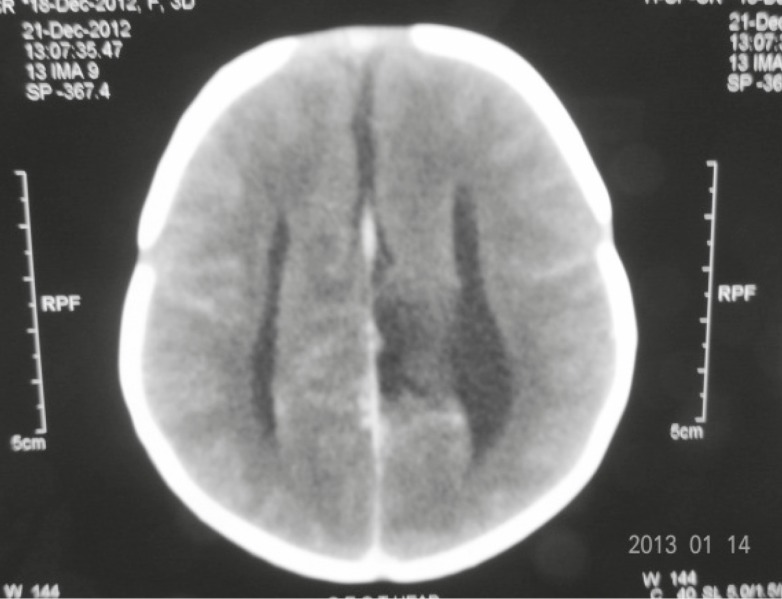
Axial CT scan showing parallel ventricles
